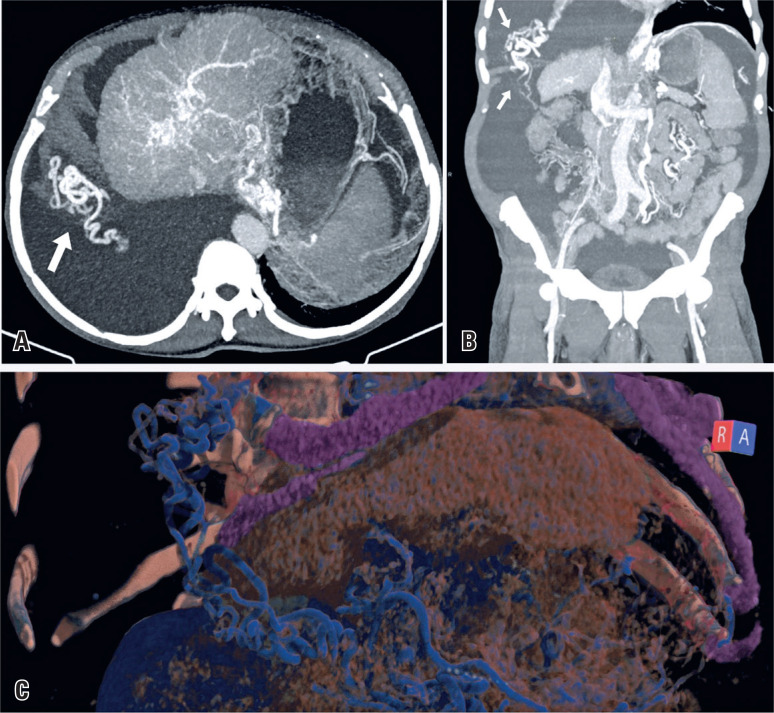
Contrast-enhanced computed tomography reconstruction images in the portal venous phase of a 70-year-old man diagnosed with liver cirrhosis. (A) Axial and (B) coronal maximum intensity projection images reveal signs of chronic liver disease, ascites, and a large pleural effusion in the right hemithorax with vessels piercing through the right hemidiaphragm (white arrows), communicating with the pulmonary circulation. (C) Tridimensional reconstruction showing the collateral vessels ascending through the right hemidiaphragm
